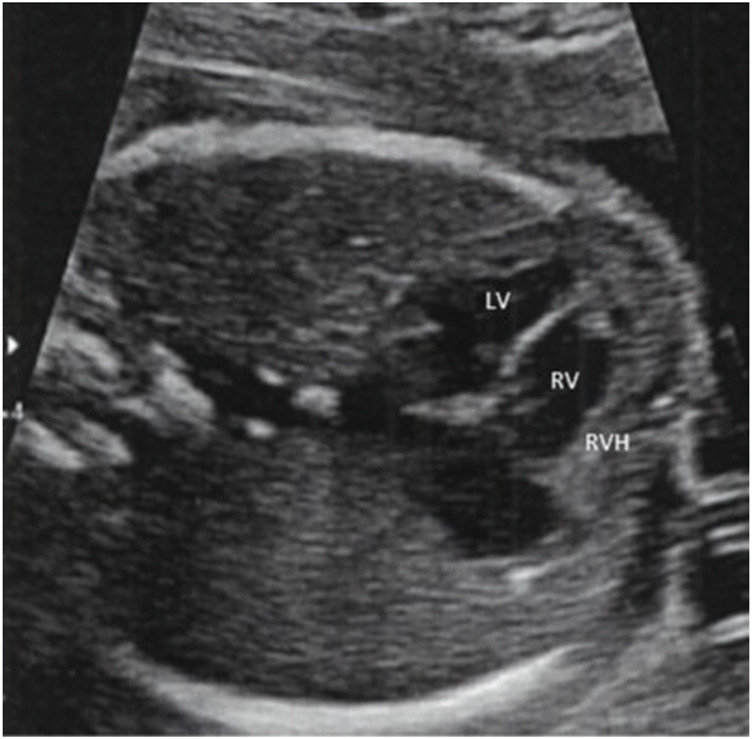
Apical 4 chamber during fetal echocardiography at 32 weeks of gestation. Cardiac ventricular asymmetry and right ventricle cardiac hypertrophy. LV: Left Ventricle; RV: Right Ventricle; RVH: Right Ventricle Hypertrophy.
